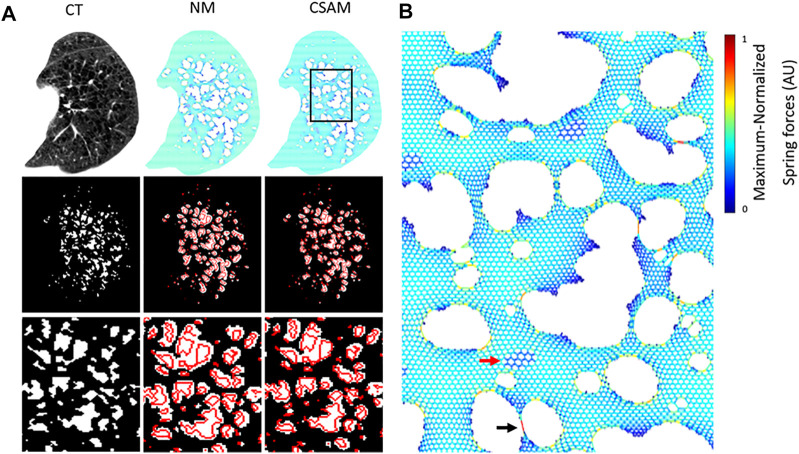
(A) Original CT from a patient and examples of mapping to spring networks using the naïve method (NM) and the Curvature and Size Adjusted Method (CSAM). The left column displays the CT scan of an emphysema patient (top), the binary low attenuation area (LAA) map obtained by thresholding at −960 Hounsfield Unit (middle), and a zoomed-in region of the LAA map (bottom). The middle and right columns represent the same content for two spring networks constructed from the CT image with the NM and CSAM. The red lines represent the boundaries of LAA clusters on the original CT. (B) Zoomed-in version of the rectangle on the CSAM network in (A). Colors are proportional to force. The black arrow points to narrow bridge carrying a high force whereas the red arrow marks a region of high attenuation.
